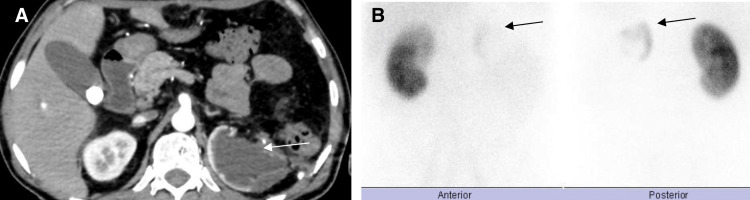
(A) Axial contrast-enhanced CT scan of the abdomen shows remnant left kidney tissue (arrow). (B) DMSA renal scan demonstrates radiotracer uptake at the left upper renal region (arrows). CT: computed tomography; DMSA: dimercaptosuccinic acid
